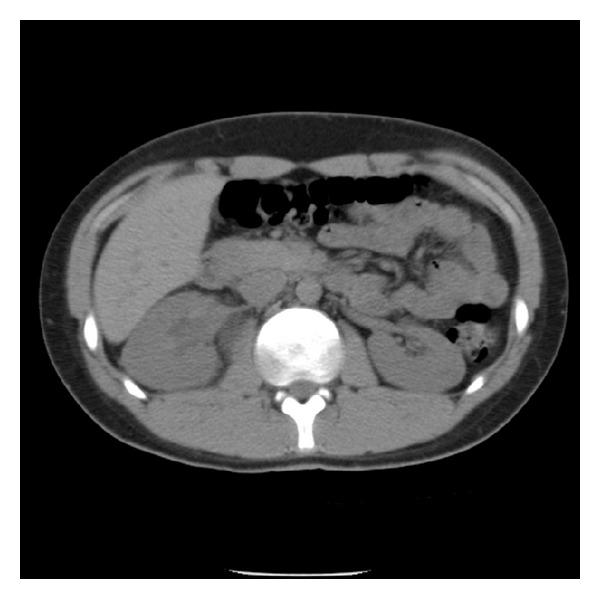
Axial CT image demonstrating nonspecific soft tissue density along the right perinephric space without hydronephrosis.
